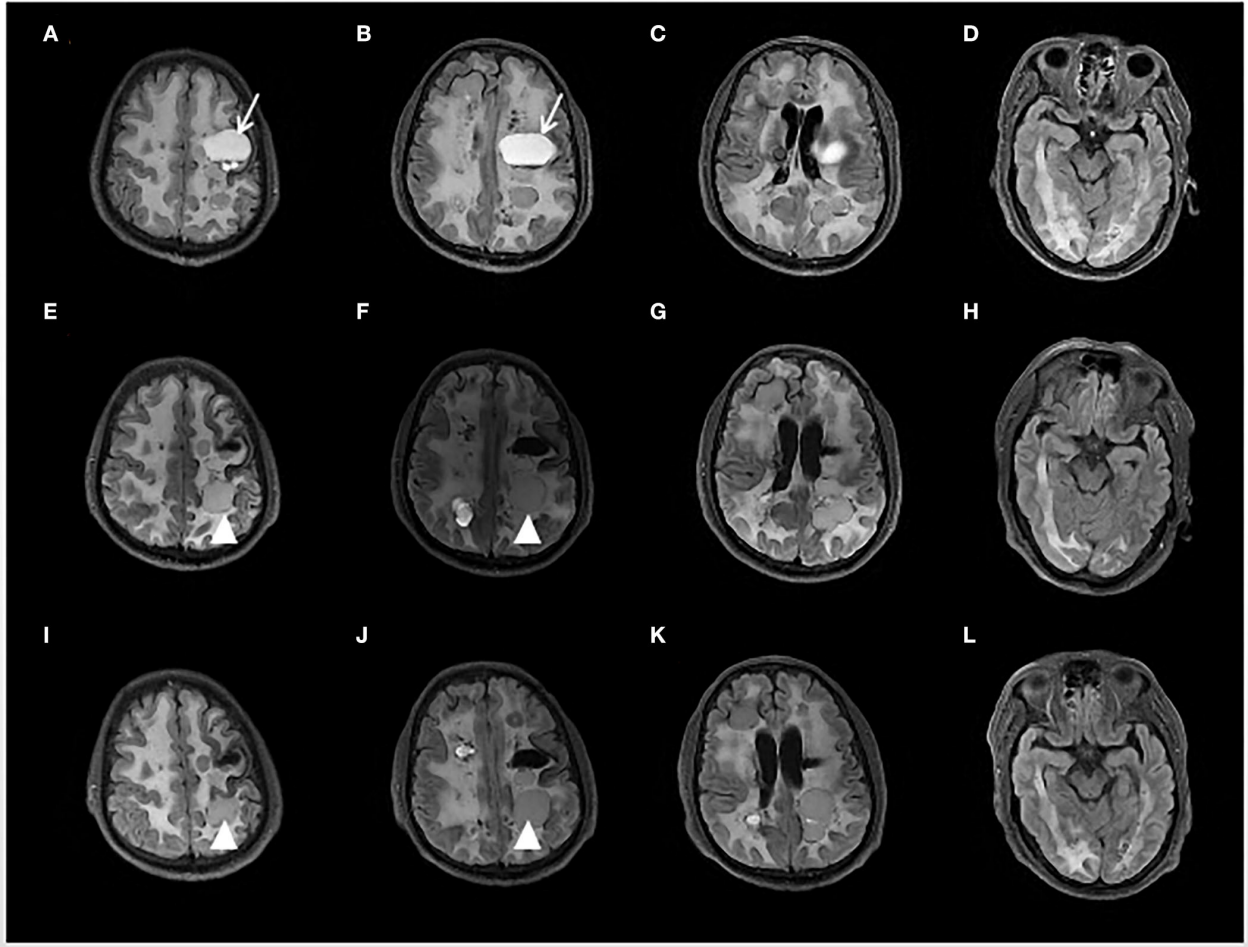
Brain fluid-attenuated inversion recovery (FLAIR) sequence of initial presentation (A–D), 6 months (E–H), and 12 months (I–L) after Bevacizumab treatment. The initial MRI showed multiple cystic foci and white matter lesions in bilateral cerebral hemispheres. The left mass cystic foci [showed as an arrow in (A,B)] was decompressed by an Ommaya reservoir. After 6 months treatment of Bevacizumab, another left frontal lobe cystic foci [showed as an arrowhead in (E,F,I,J)] was slightly enlarged.
